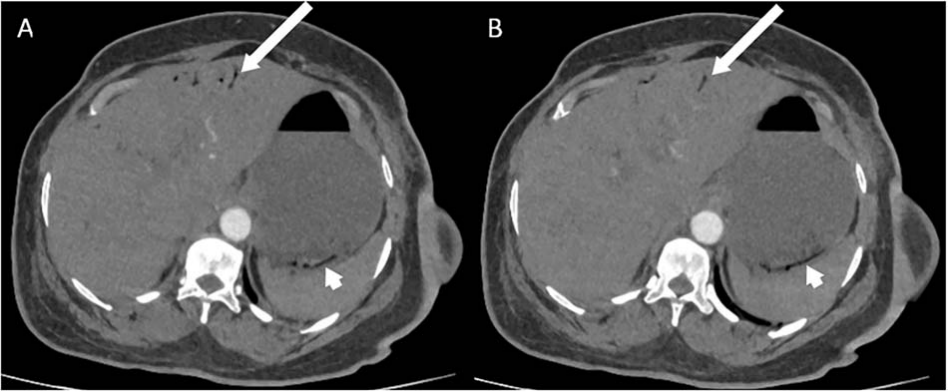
CT pulmonary angiogram images demonstrating moderate amount of branching linear gas in the liver (long arrows), most pronounced in (A) at the level of T11/12; gas within the wall of the gastric fundus (short arrows) can be appreciated more in (B) at the level of T11.
